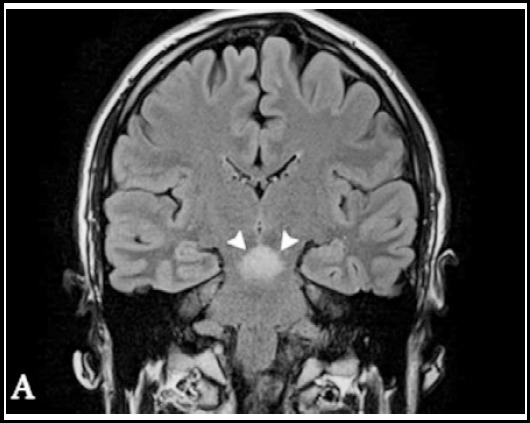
Coronal inversion recovery MR image shows mesencephalic lesion with oedemaas a hyperintense area (arrowheads).
